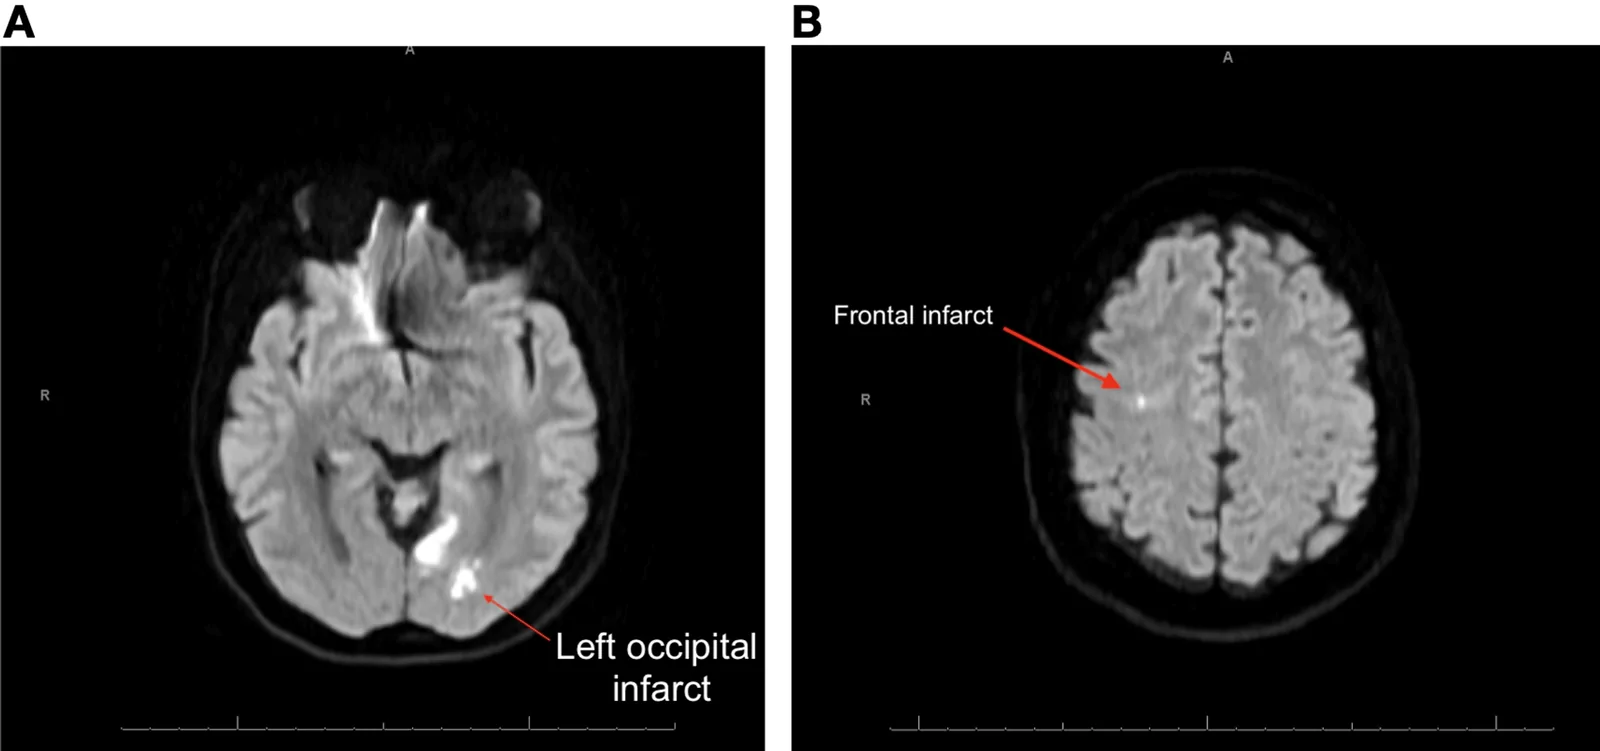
Brain magnetic resonance imaging MRI demonstrating multiterritory embolic infarctions MRI: magnetic resonance imaging MRI of the brain without contrast. (A) Axial diffusion-weighted imaging (DWI) demonstrating a focal area of acute hyperintense signal within the left occipital lobe corresponding to an acute ischemic infarct. (B) Separate axial sequence slice demonstrating a small acute ischemic focus bordering a chronic, remote infarct within the right frontal lobe. The presence of multiterritory cortical lesions of varying chronologies strongly indicates a recurrent central embolic etiology
